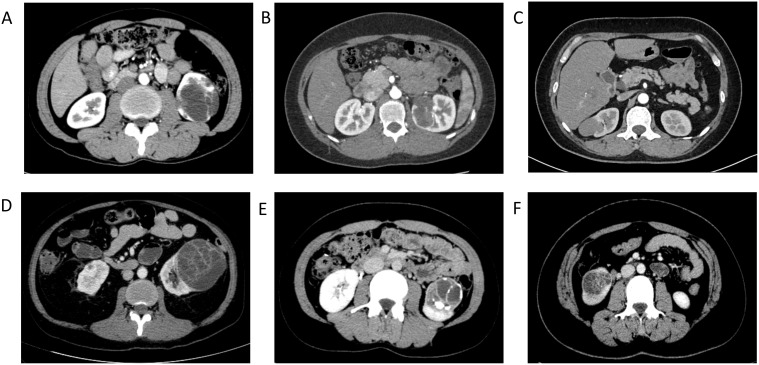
[Contrast-enhanced CT of the kidneys; (A–F) correspond to cases 1–6]: CT scans indicate that single or multiple oval-shaped low-density lesions are present within the kidneys; in 4 patients (A, D–F), septum-like high-density shadows are visible within the lesions, and upon enhancement, the linear septa show significant enhancement;.
